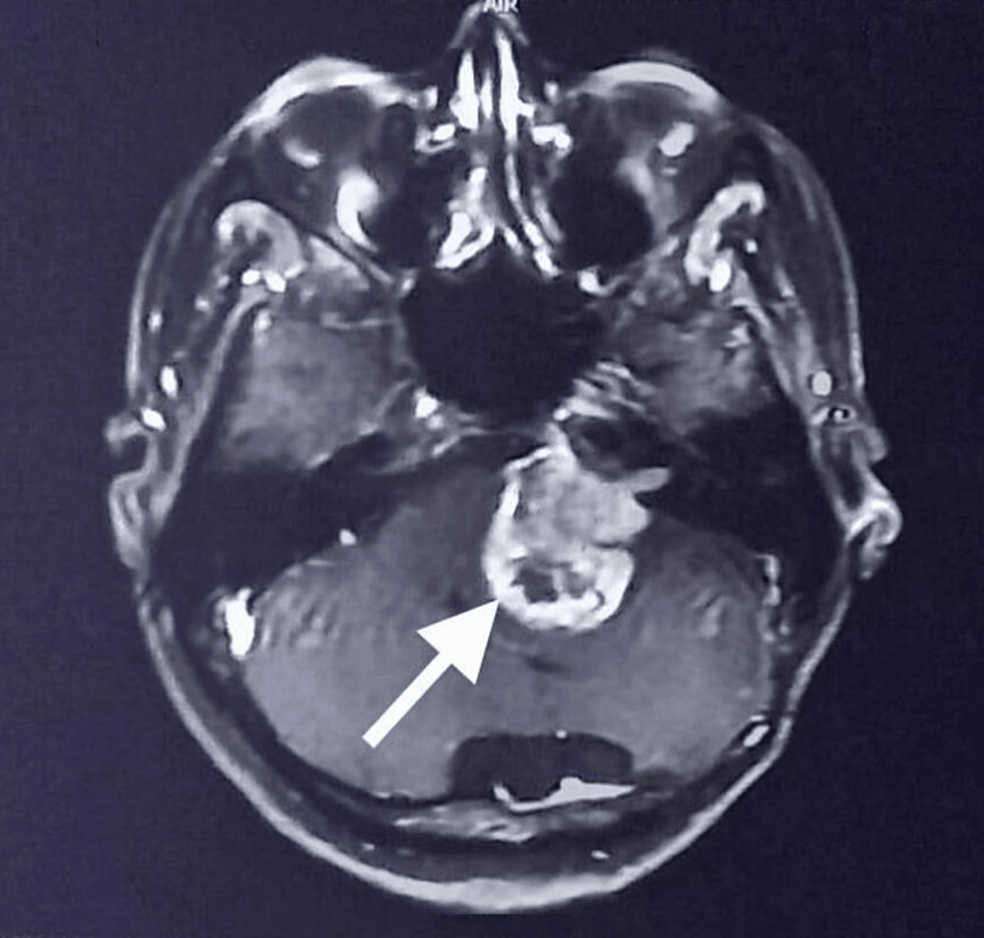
MRI of the brain showing regrowth of the residual tumor (depicted by arrow). A large heterogeneous enhancing lesion at the level of the internal auditory meatus.
